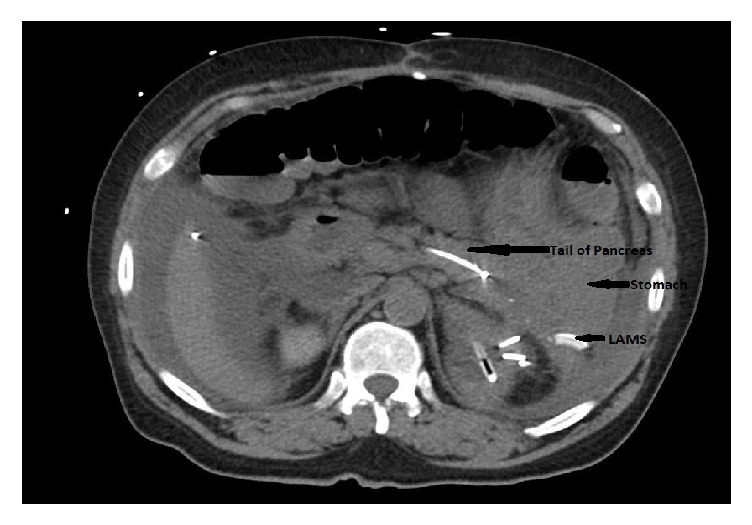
Patient 2, CT scan showing resolution of peripancreatic fluid collection.
